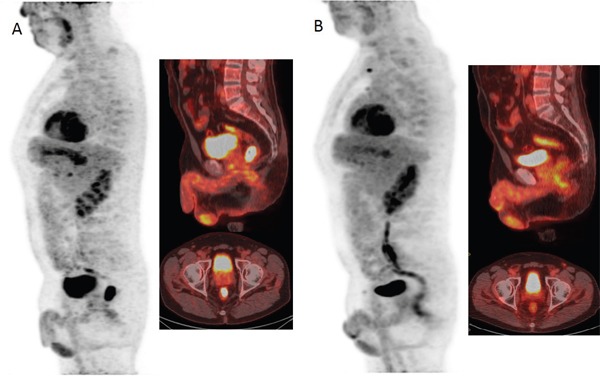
^18^F-FDG PET/CT images before A and after B treatment showed a reduction of FDG uptake with a ΔSUVmax of 96.67% classifying the patient as responder.
